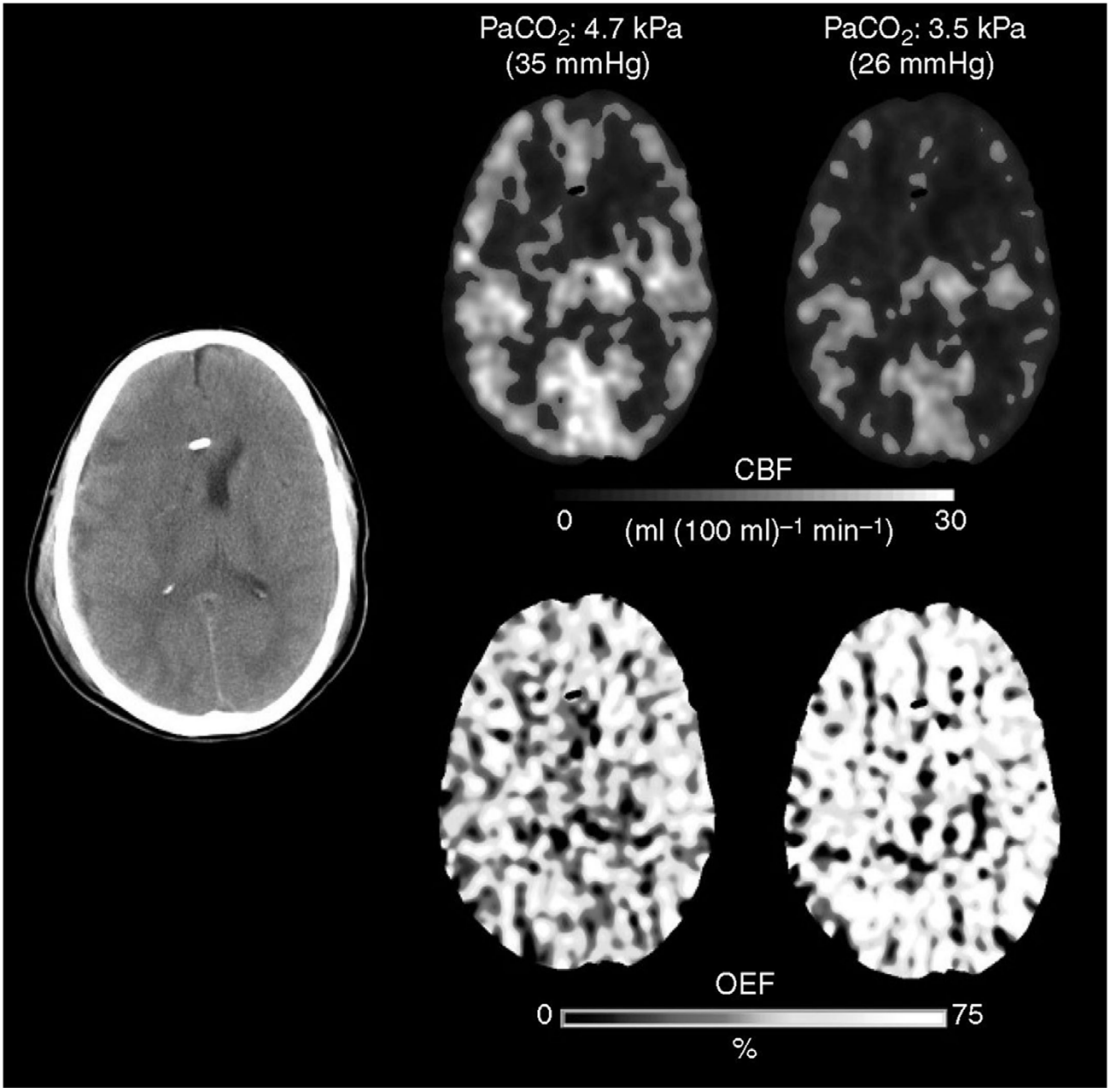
The Xenon CT imaging that showed cerebral blood flow (CBF) decrease and O_2_ extraction fraction (OEF) increase after hyperventilation.
